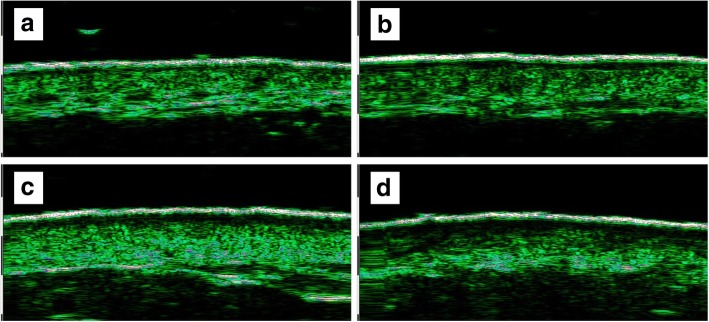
Ultrasound image of re-epithelialized skin after partial-thickness burns according to healing time (re-epithelialization time). a Healing time was 11 days, and the proportion of low-echogenic band (LEB) thickness was 14%. b Healing time was 16 days, and the proportion of LEB thickness was 20%. c Healing time was 19 days, and the proportion of LEB thickness was 25%. d Healing time was 26 days, and the proportion of LEB thickness was 30%
